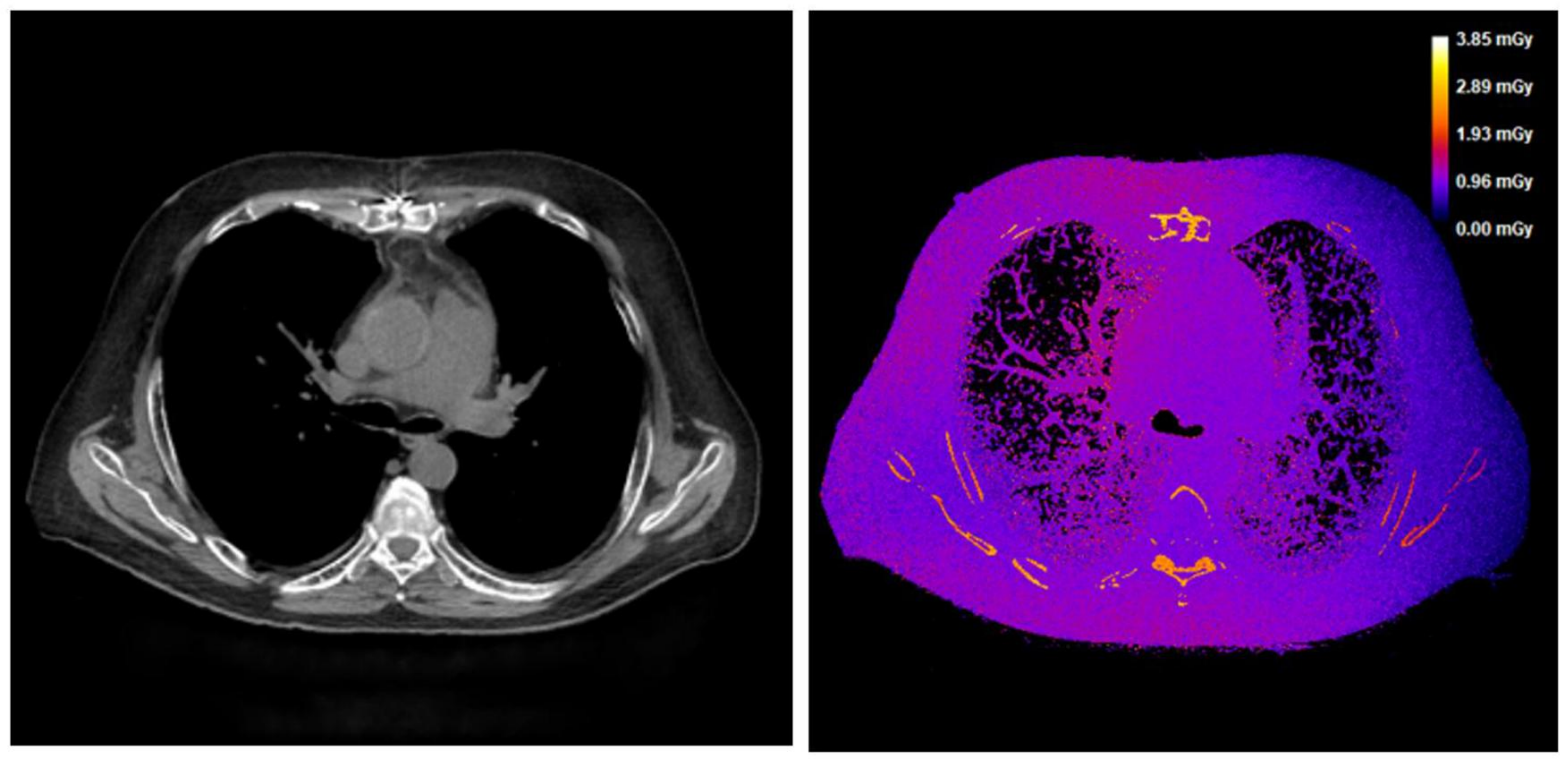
Chest CT scan (left) and corresponding dose image (right) depicting radiation dose distribution.
